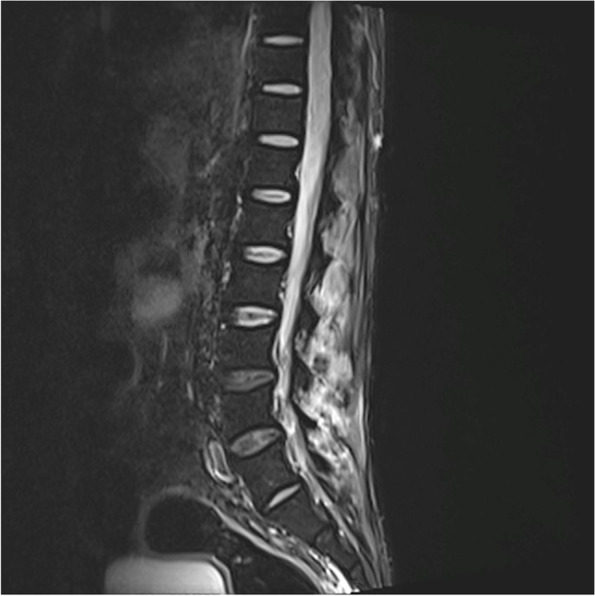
T2W fat suppressed MRI spine shows continuation of the T2 high signal up to the conus medullaris
